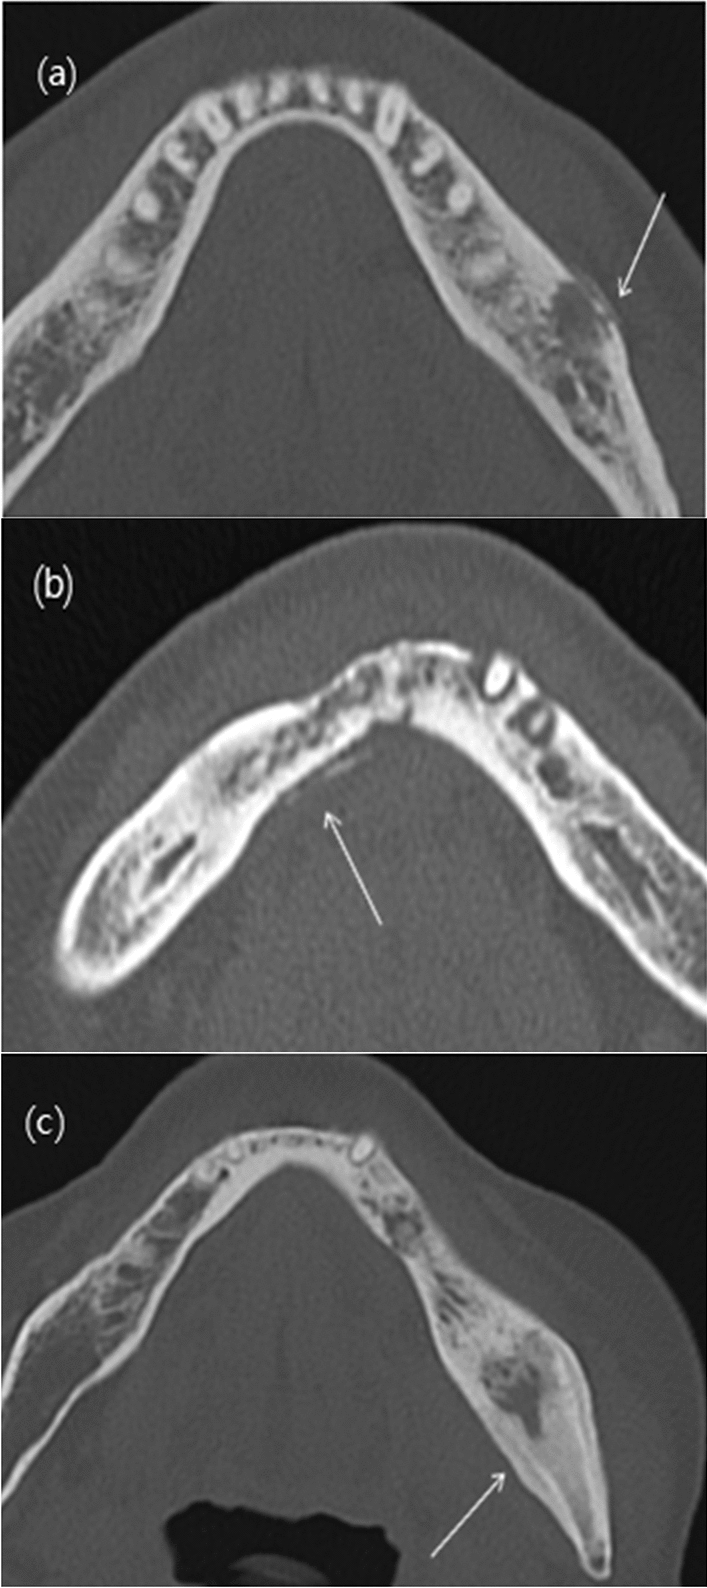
Patterns of periosteal new bone formation. Computed tomography images obtained with the bone window setting depict three types of periosteal new bone formation on the related cortical bone surface: (a) continuous lamellar, (b) interrupted lamellar, and (c) solid type.
